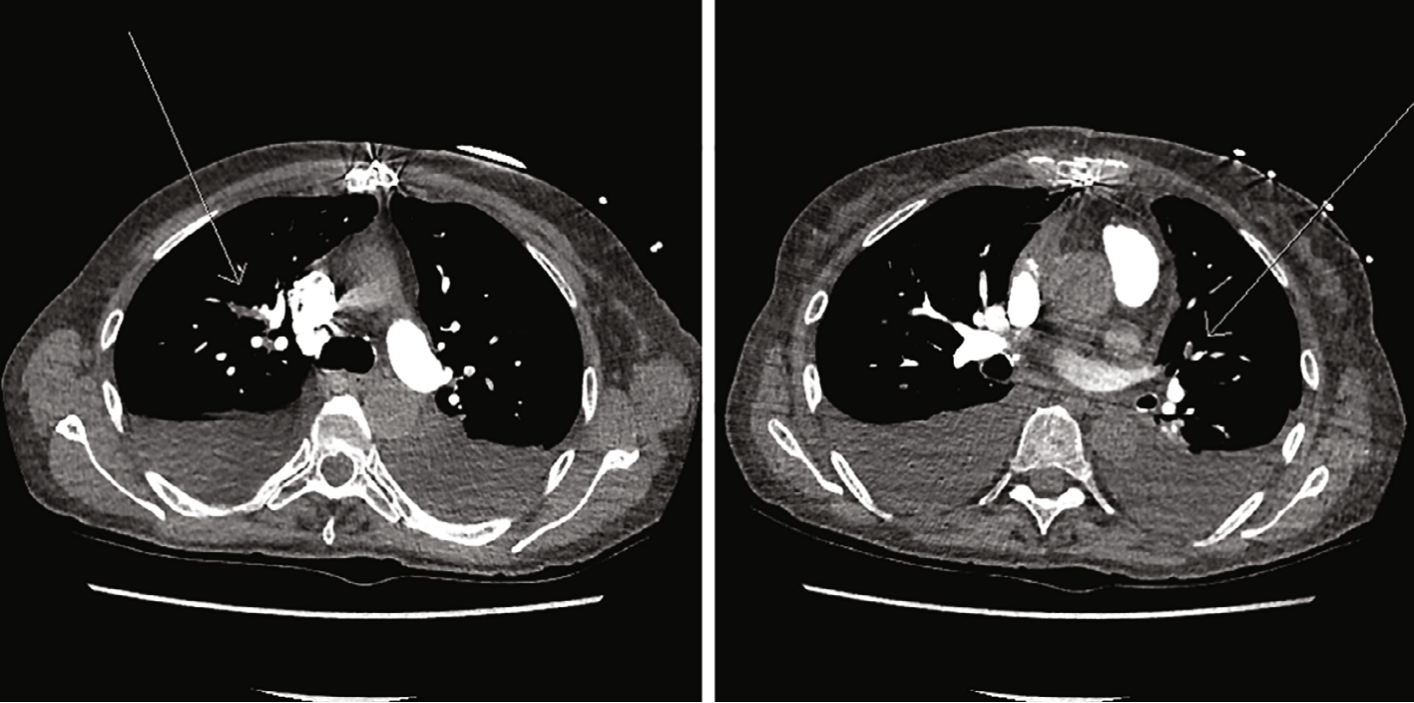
CT angiogram of the chest showing bilateral pulmonary emboli.
